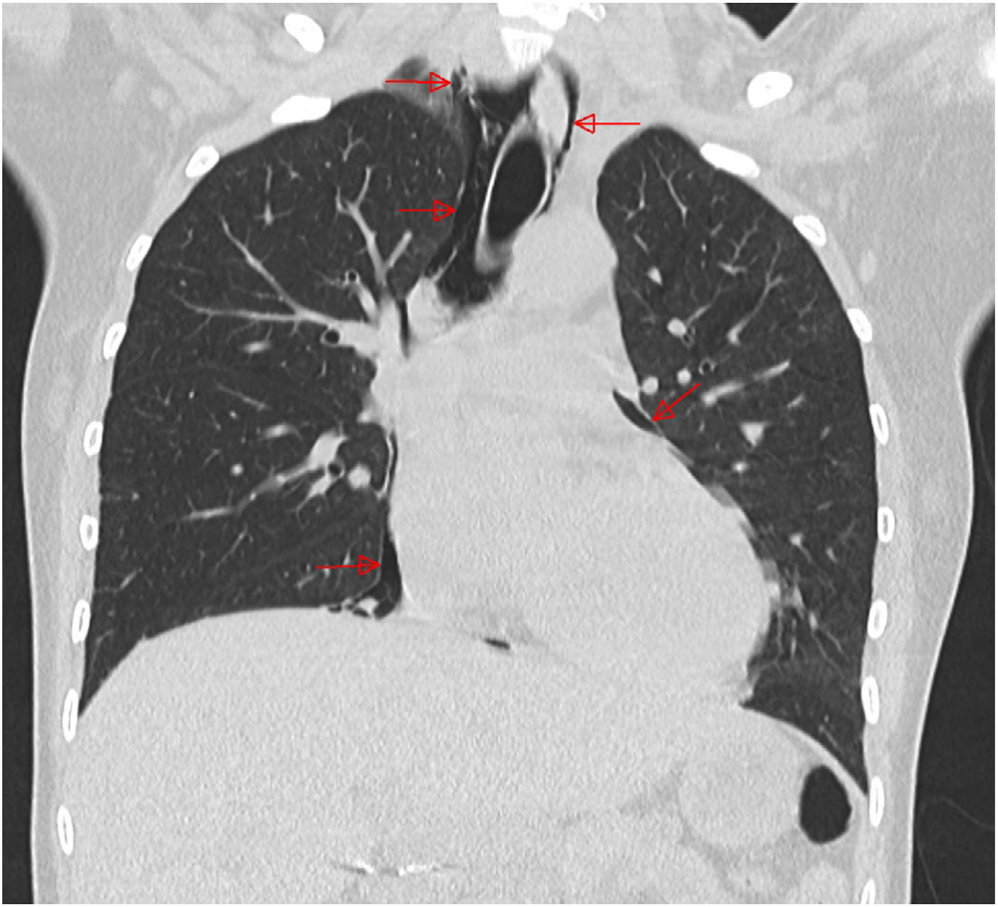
CT scanner showing Spontaneous pneumomediastin with no underlying lung lesion in patient 3 with severe SS sickle cell disease at presentation
